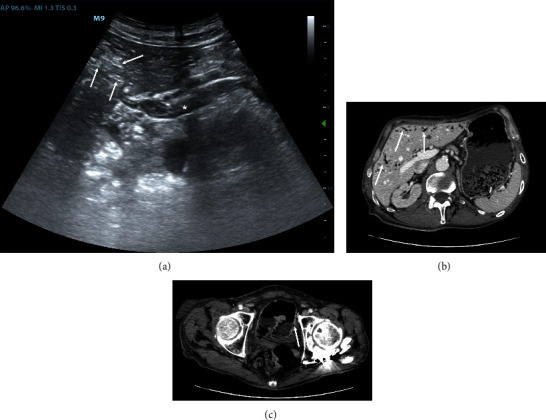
POCUS and CTA of case 1. (a) POCUS shows portal vein at hepatic hilum (asterisk) with multiple hyperechogenic spots into the lumen. White arrows indicate air in intrahepatic portal vessels with altered liver ultrasonographic structure. (b) A CTA scan confirms air into the liver (white arrows), while air is not easily identified into portal vein. (c) CTA confirms a little area of pneumatosis intestinalis (white arrow). POCUS stands for point-of-care ultrasonography; CTA stands for computed tomographic angiography.
